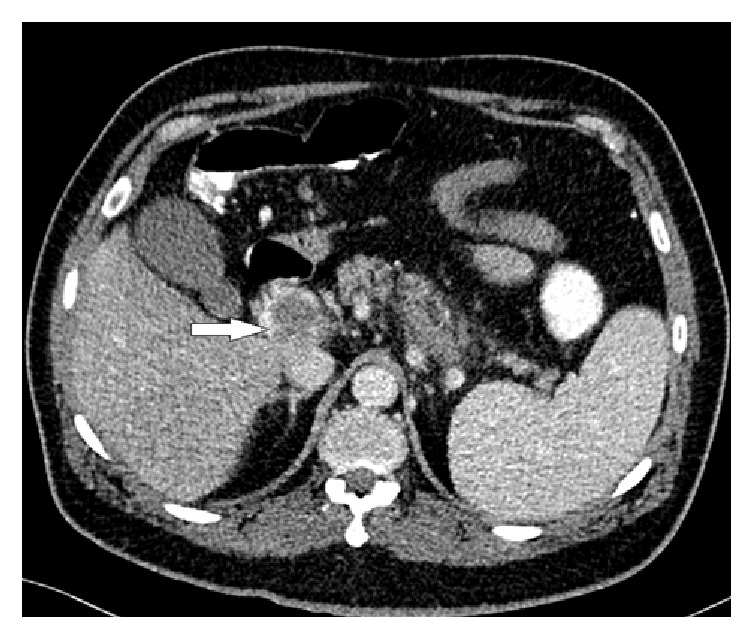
Abdomen CT scan with contrast (axial image) shows distended portal vein with filling defect, indicating thrombosis (white arrow).
